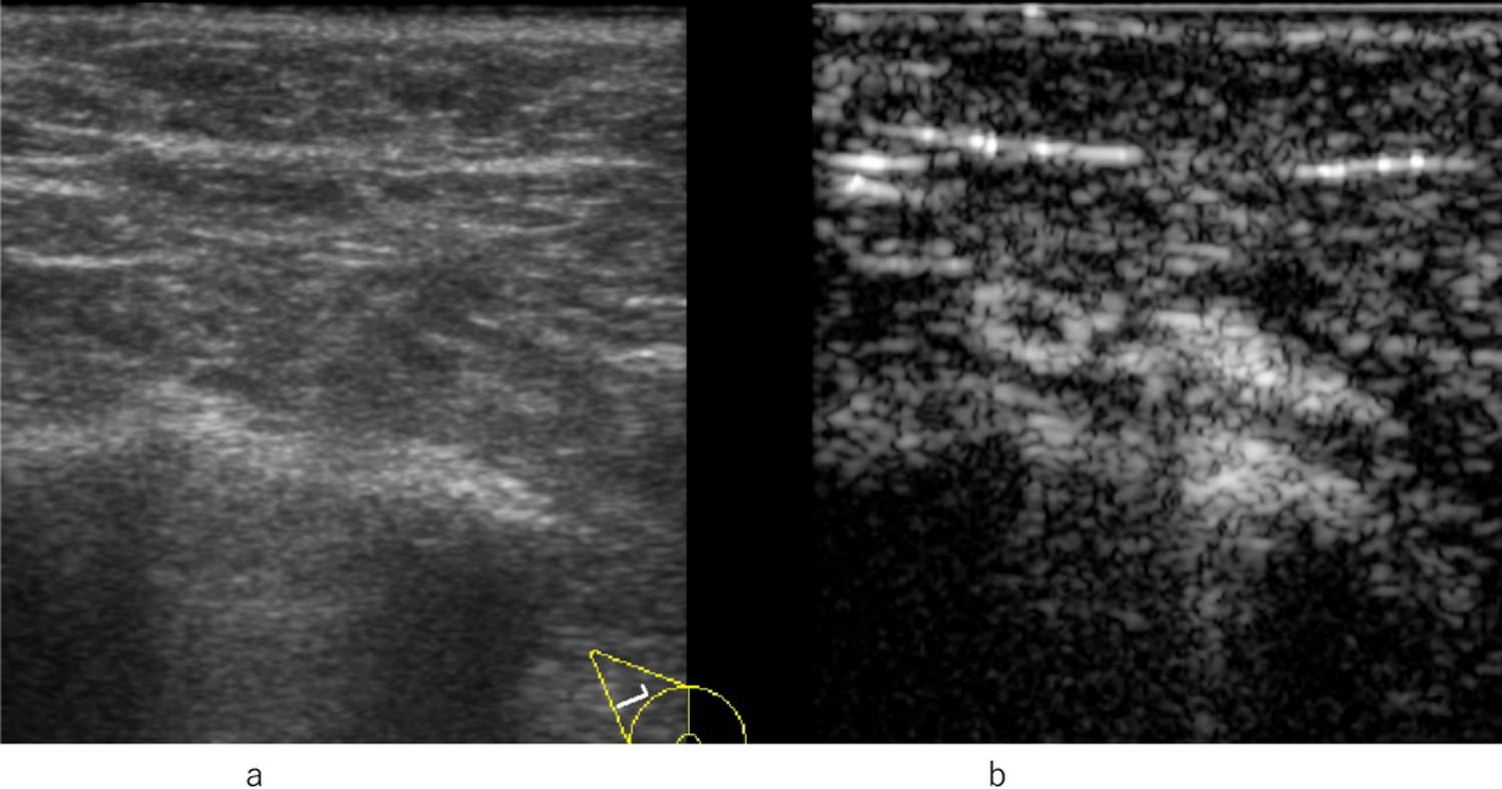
Conventional ultrasound (US) and lymphatic contrast-enhanced ultrasound (CEUS). a Conventional US shows a sentinel lymph node (arrow). b An oval-shaped contrast-enhanced lymph node (arrow) is visualized within a few minutes after Sonazoid injection
